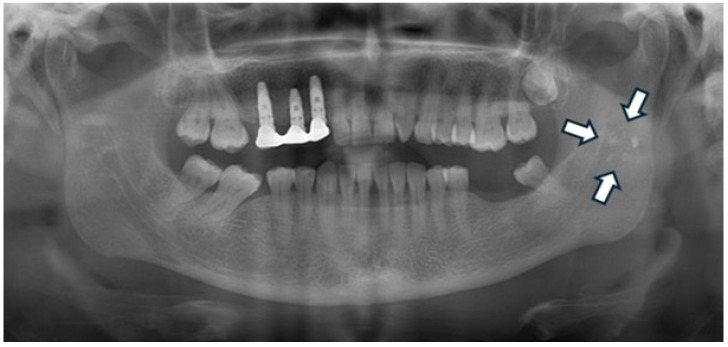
Panoramic radiograph of a 36-year-old male patient showing a well-defined radiopaque mass superimposed over the left mandibular ramus region, consistent with a tonsillolith.
